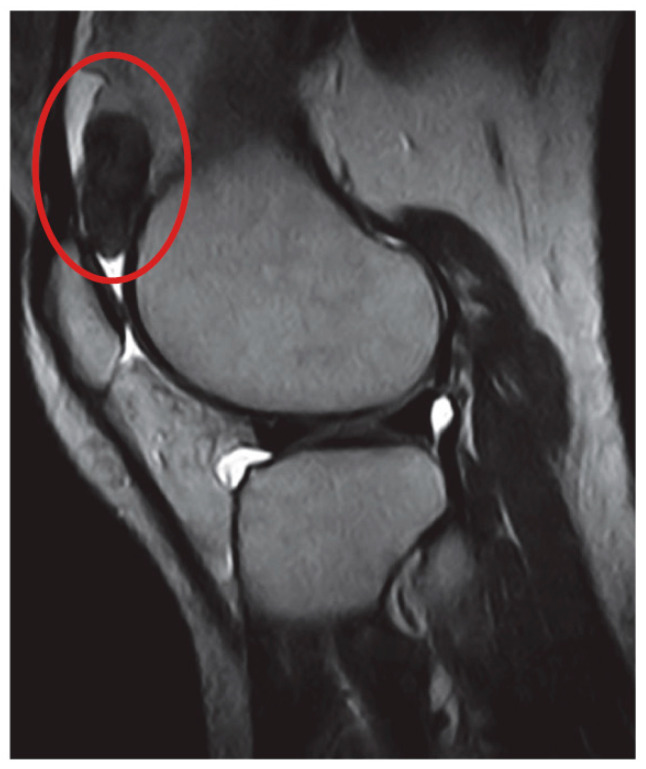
T2-weighted magnetic resonance sagittal scan of L-TGCT. The location of disease is indicated by a red circle.
